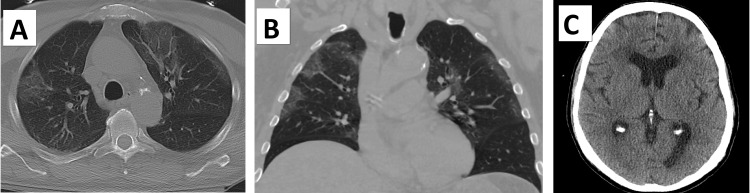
Postoperative imaging. (A) Representative axial and (B) coronal views of a thoracic CT scan demonstrating airspace infiltrates and ground-glass opacities in both upper lobes and the right middle lobe, with no evidence of a discrete tumor; and (C) representative axial CT scan of the brain with no evidence of metastasis to the brain.
